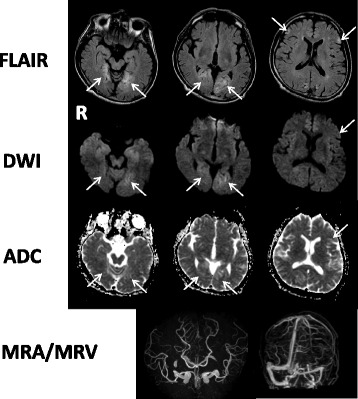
Fluid-attenuated inversion recovery (FLAIR) images and diffusion-weighted images (DWI) show multiple high-intensity signals (arrows) in the cortical and subcortical areas of the bilateral occipital and the mesial temporal lobes, and the left frontal lobe. Apparent diffusion coefficient (ADC) images show normal- to slightly low-intensity signals in the areas with the abnormal signals shown by FLAIR and DWI (arrows). Magnetic resonance angiography and venography (MRA and MRV) show no abnormalities
